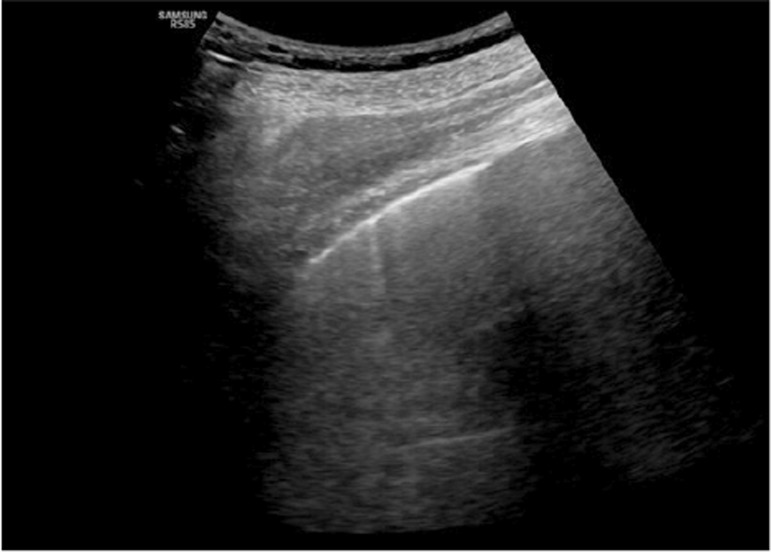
Ultrasonographic B-line artifacts: vertical lines moving in conjunction with the lung sliding from the pleural line to the screen edge and erasing the A-lines
